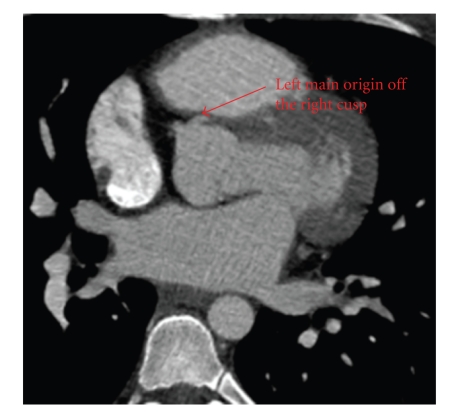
Coronary CT showing the origin of the left main (LM) from the right coronary cusp.
